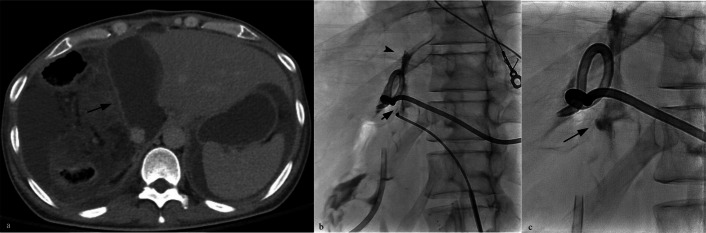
a Contrast enhanced CT abdomen demonstrate post hepatectomy biloma. b Catheterization of bile duct-biloma connection (arrow) with contrast injection filling biloma (arowhead). c Post embolization image with Obsidio embolic within connection as well as the biloma
